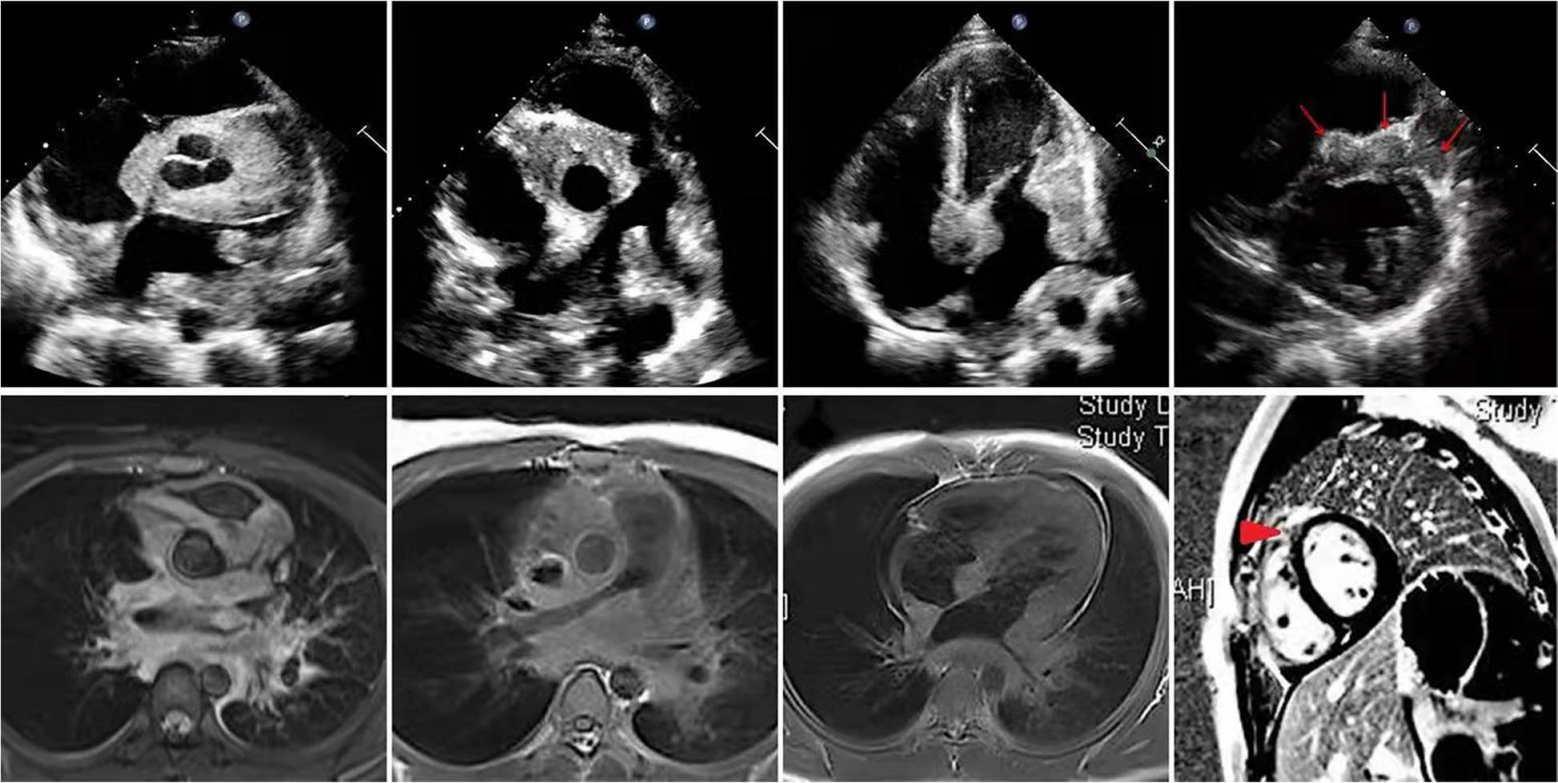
Echocardiography and enhanced cardiac MRI at admission showed diffuse lesions invading the heart and infiltration of the myocardium (arrow)
